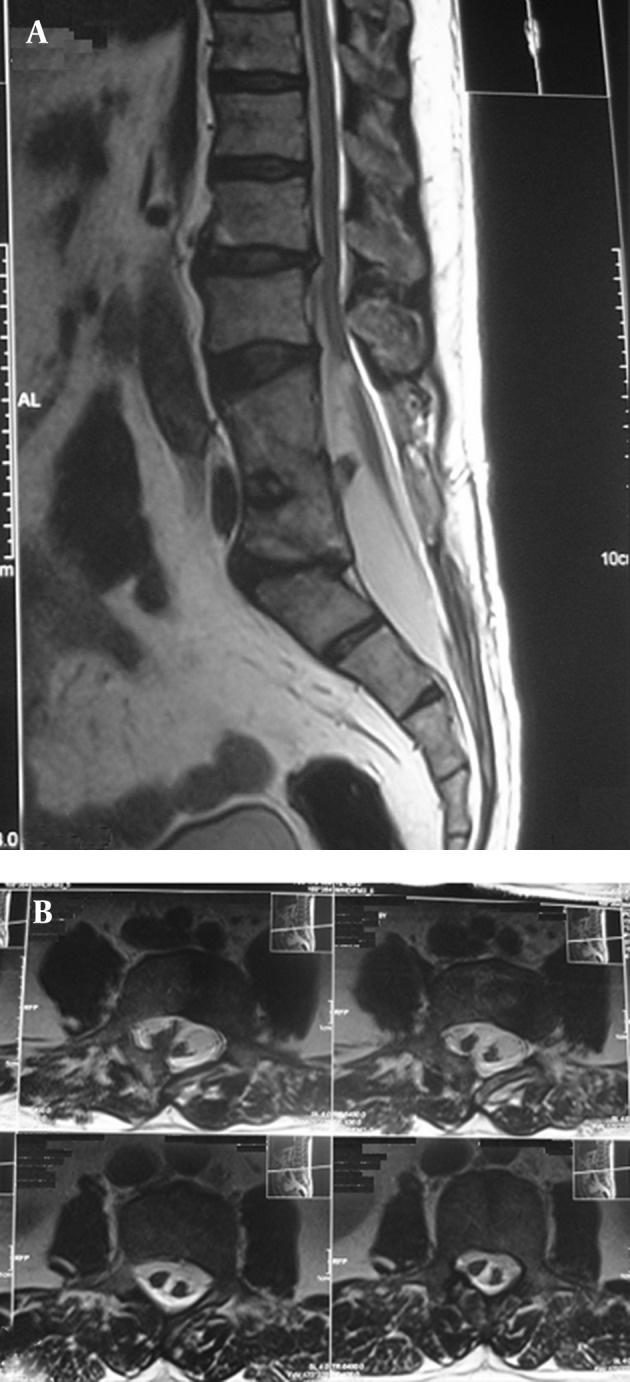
Mid-sagittal (A), and Axial (B) T2-weighted Images Showing Split Spinal Cord. The Spinal Cord is Split From Approximately L3 to L4 With Tethering of the tip of the Conus Medullaris at L5
